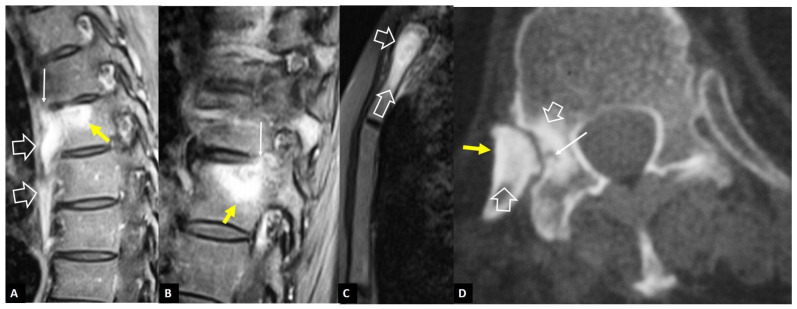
SAPHO syndrome in a 39-year-old female patient with acne, postulosis, and chronic sternal and back pain. Parasagittal fat-suppressed contrast-enhanced T1-w MR images (A,B) showing enhancing bone marrow edema in two different levels (yellow arrows) with early disc involvement (thin arrows) and soft-tissue inflammatory changes anterior to the thoracic spine (open arrows). (C) Sagittal STIR MR image showing the bone marrow edema in the manubrium of the sternum in keeping with osteitis (open arrows). (D) Axial CT showing hyperostosis of the right rib (yellow arrow), osteitis (open arrows), and erosion (thin arrow).
